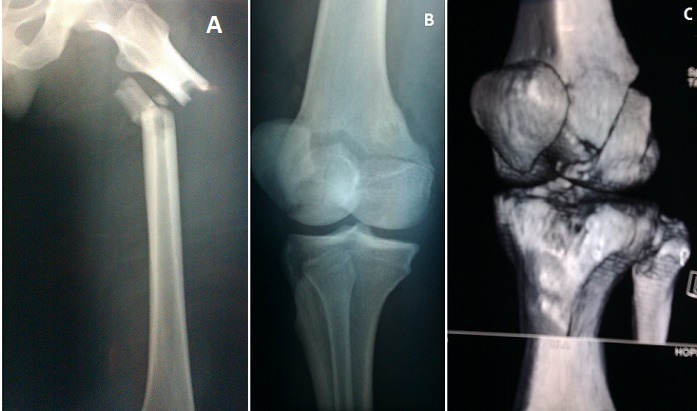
(A) radiographie standard du fémur objectivant une fracture du 1/3 supérieure de la diaphyse fémorale; (B) incidence de ¾ du genou montrant la fracture de Hoffa; (C) TDM avec reconstruction 3D montrant mieux la fracture de Hoffa
